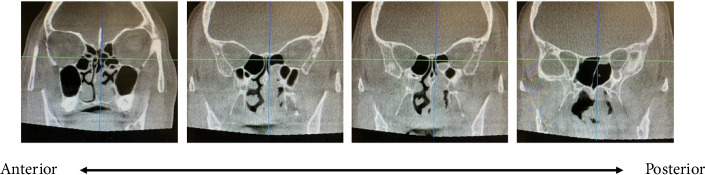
Computed tomographic scan of nasal mass prior to endoscopic resection.
